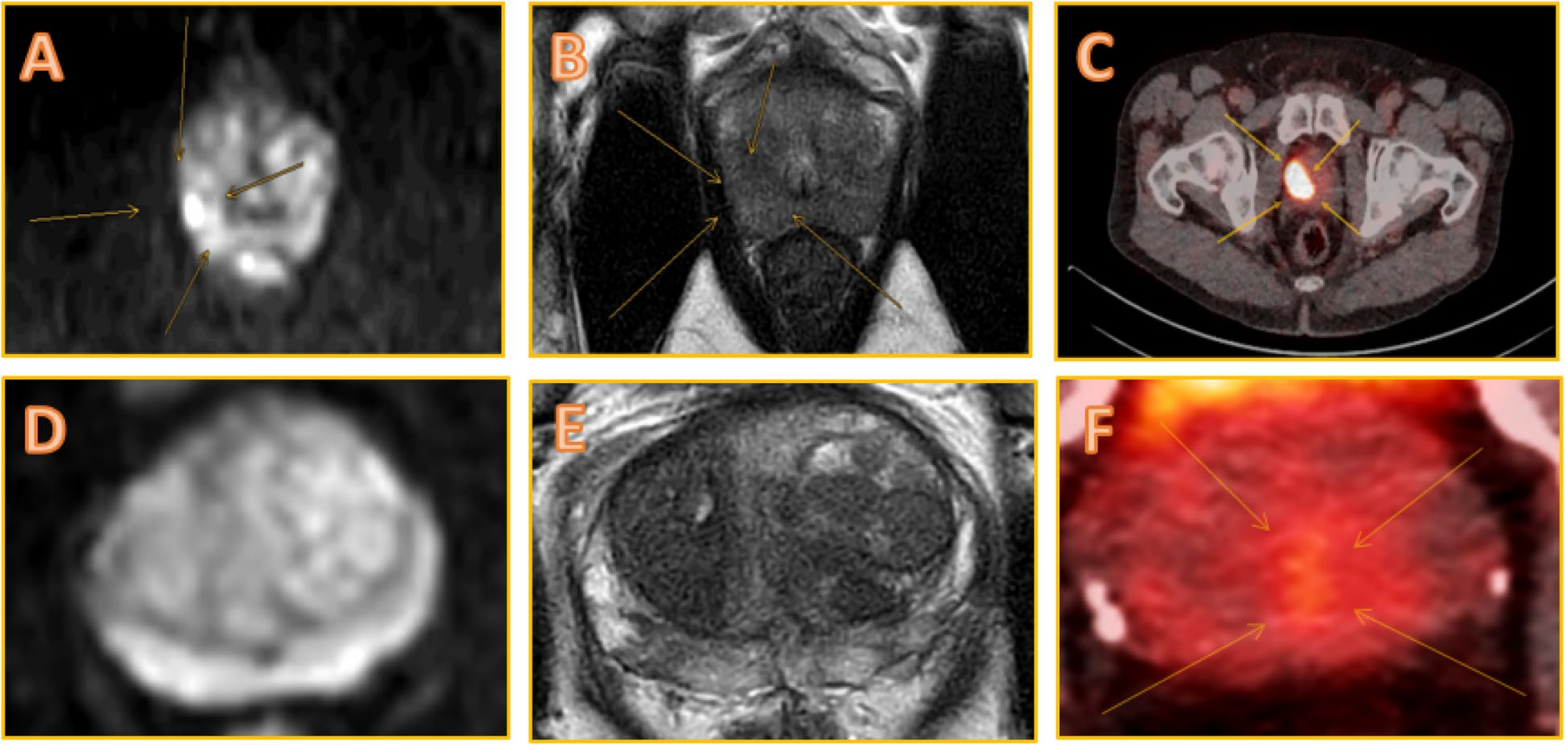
Annotation of suspicious lesions on imaging. A–C Concordant positive findings of clinically significant prostate cancer (csPCA) in the peripheral zone of the right prostate lobe on multiparametric magnetic resonance imaging (MRI) and gallium-68 prostate-specific membrane antigen positron emission tomography/computed tomography (PSMA-PET/CT). In the DEPROMP Trial, uro-radiologists (A, B) and nuclear medicine physicians (C) annotate defined suspicious lesions in imaging layers to enable effective and clear interdisciplinary communication before targeted biopsy (TB) as demonstrated in exemplary fashion. An example of a false-negative MRI with true-positive PSMA-PET/CT in the posterior transitional zone of the left prostate lobe is shown in series D–F. The PSMA-PET/CT-guided TB detected a solitary, Gleason 3 + 4 PCA. In both cases, radical prostatectomy confirmed the risk stratification based on biopsy yields
